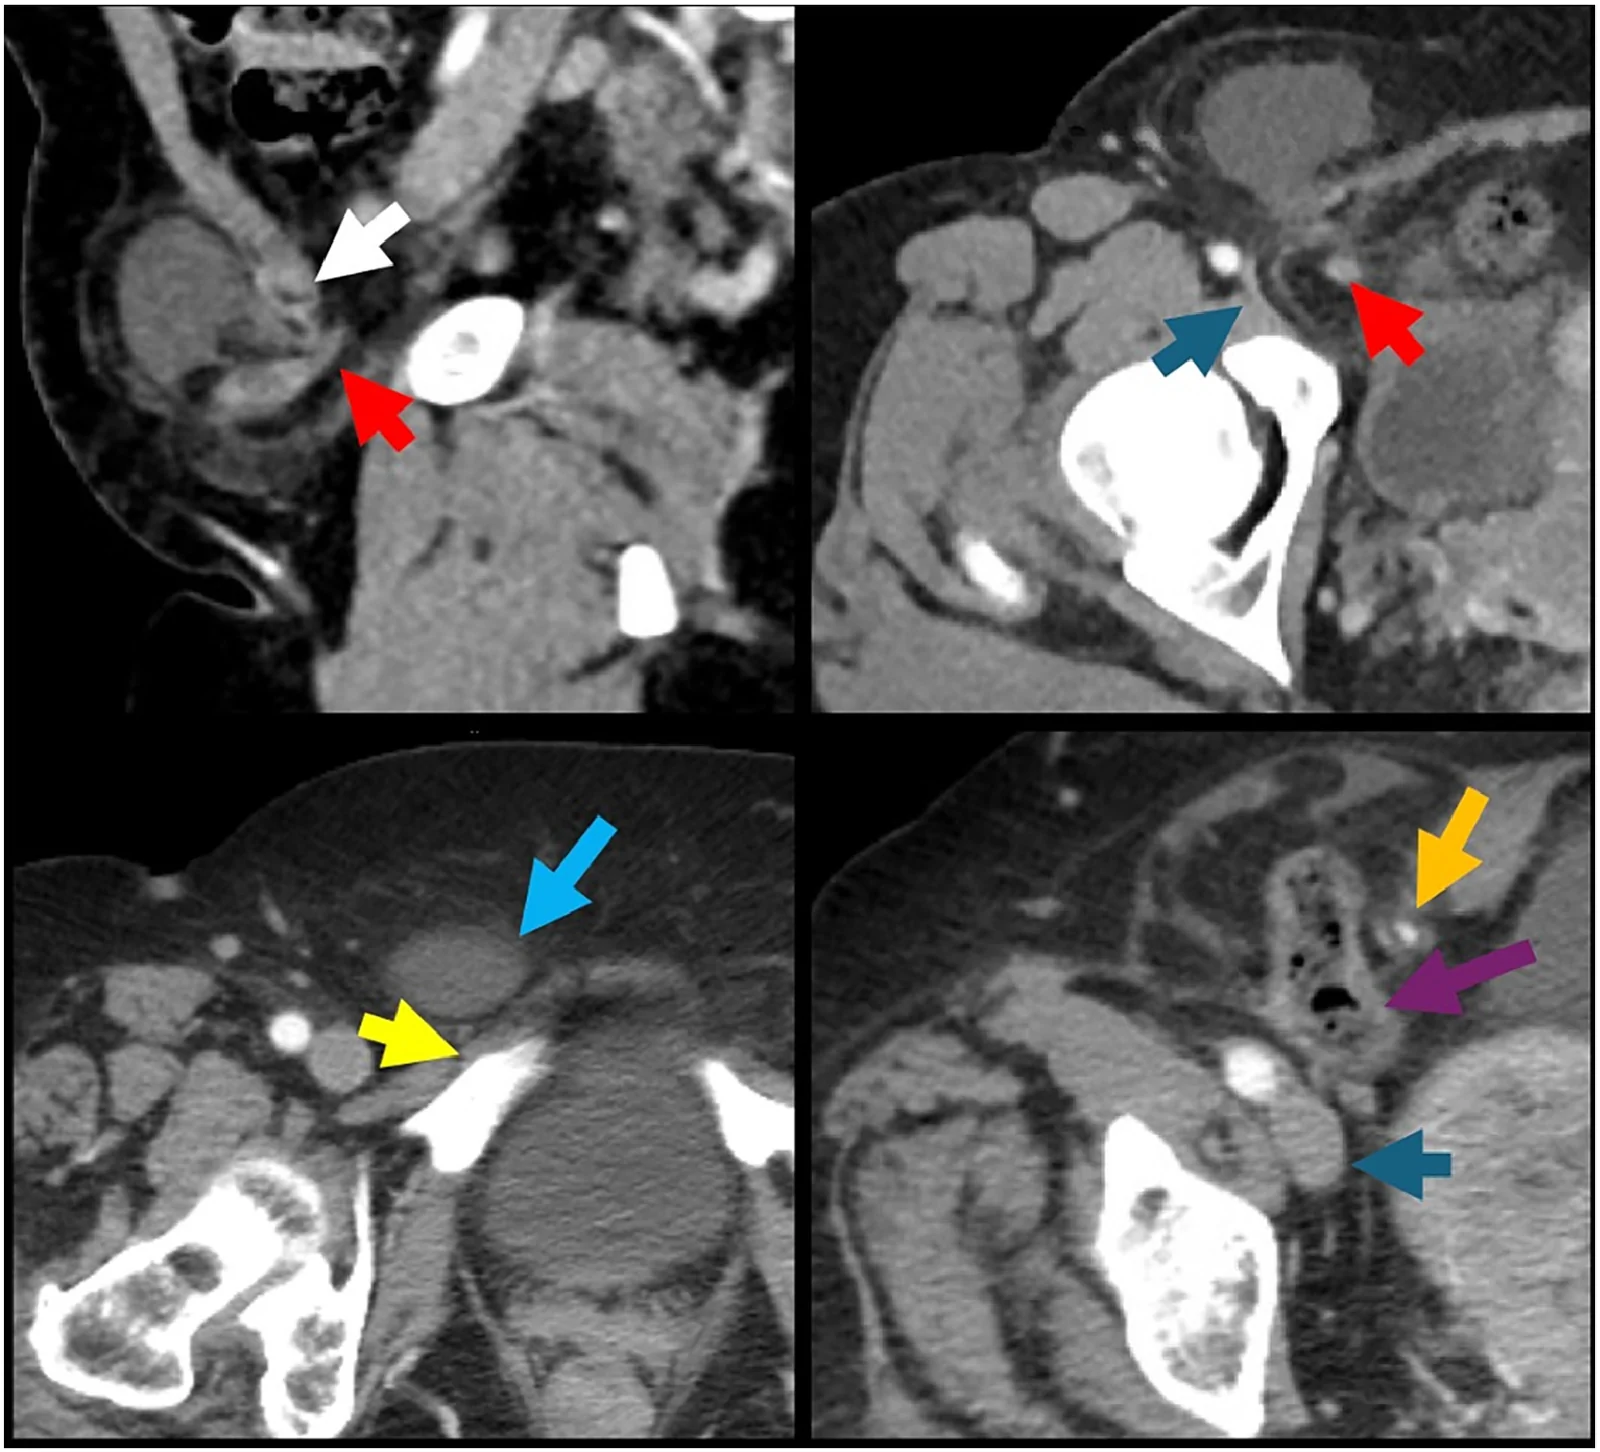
Clockwise from top left: Sagittal CT image demonstrates herniation of the appendix (red arrows) through a femoral hernia below the abdominal wall (white arrow). Axial CT image of the same patient showing herniation of the appendix (red arrow) through a femoral hernia medial to the femoral vein, which is compressed (blue arrow). Axial CT image of a different patient demonstrating herniation of a bowel loop (purple arrow) through an inguinal hernia lateral to the inferior epigastric vessels (orange arrow); there is no compression of the femoral vein at the level of the hernia neck (blue arrow). Axial CT image of the same patient demonstrates the hernia sac (light blue arrow) extending medial to the pubic tubercle (yellow arrow).
